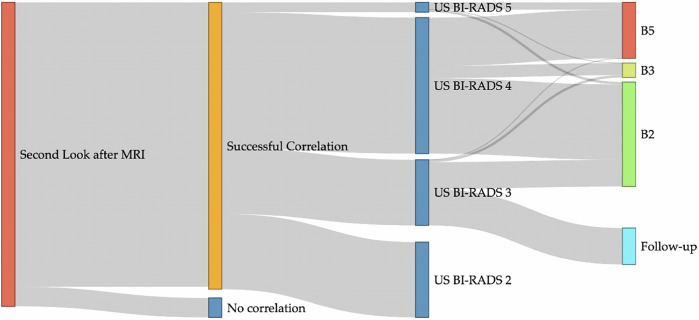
Diagnostic flow of patients undergoing second-look evaluation with real-time virtual ultrasound, including BI-RADS classification and final outcomes
